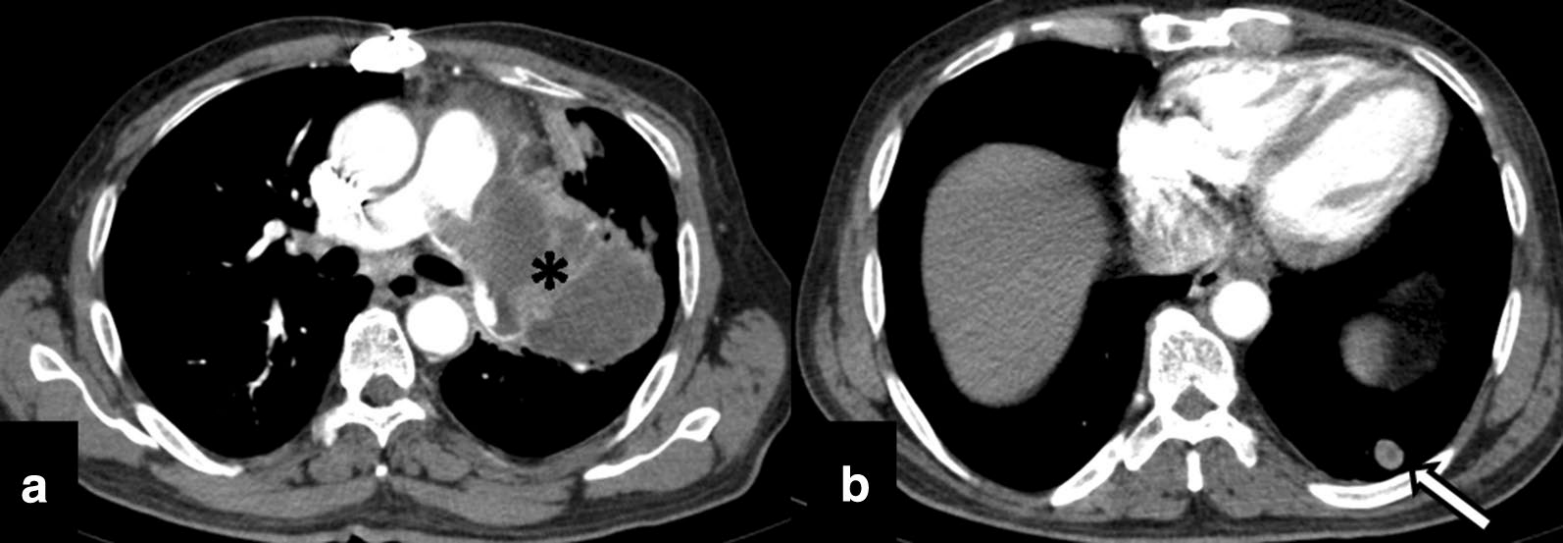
Follow-up imaging after 12 cycles of radiotherapy shows a large lobulated peripherally enhancing exophytic mediastinal mass (*asterisk*) arising from the left main pulmonary artery and invading its segmental arteries with a well-defined soft tissue nodule in left lower (*arrow*). CT findings were suggestive of disease progression with pulmonary parenchymal metastasis
